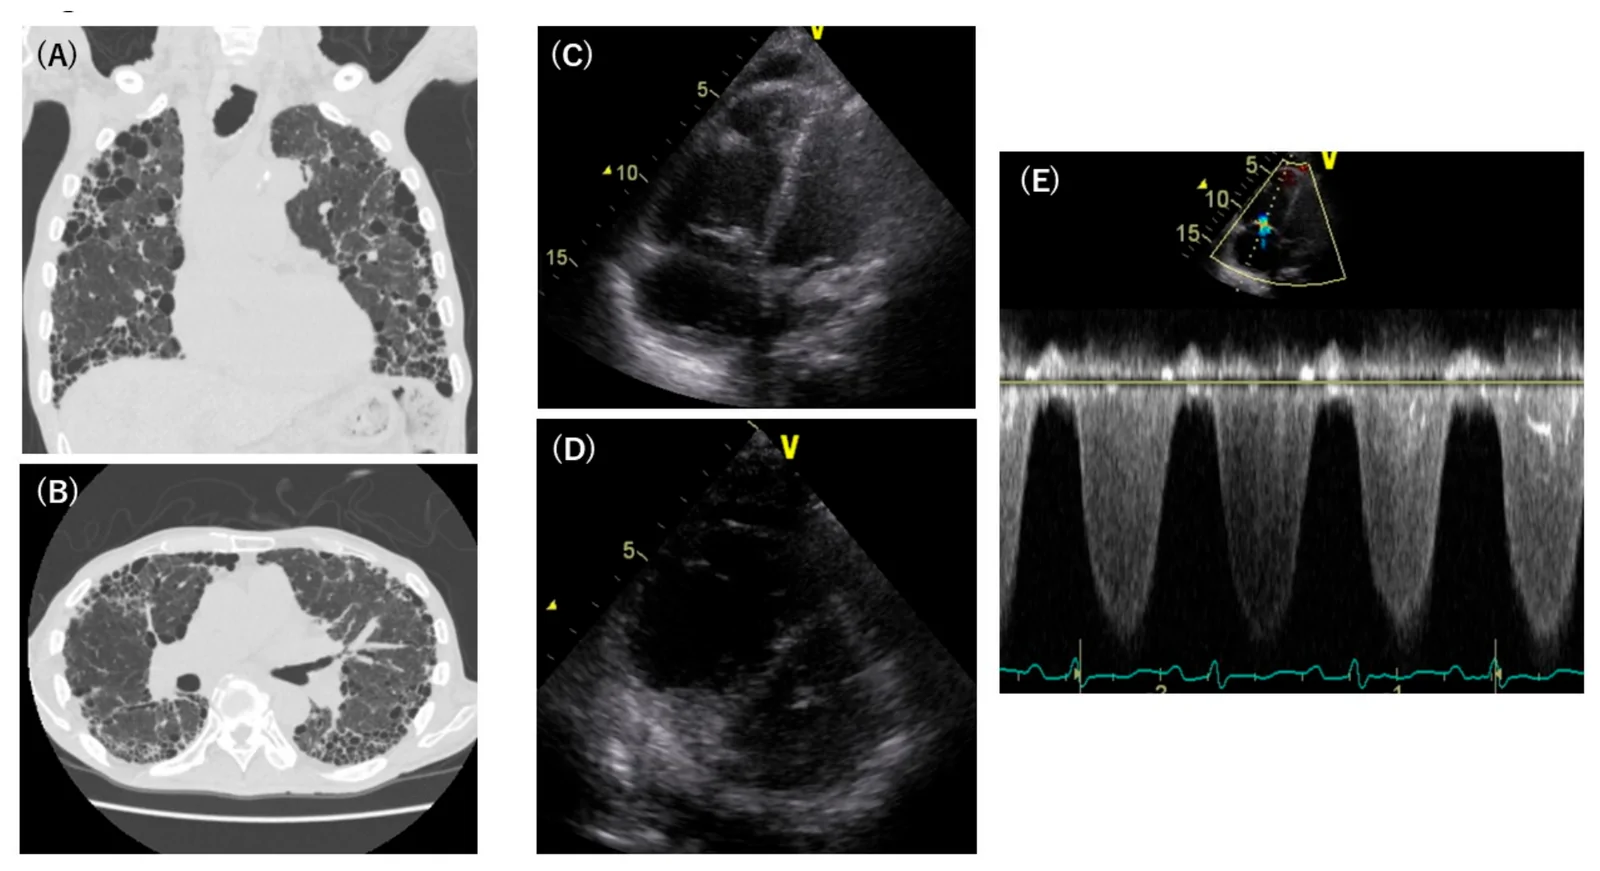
Representative chest HRCT and echocardiographic images of a patient with severe interstitial lung disease and pulmonary hypertension (ILD-PH). (A) Coronal and (B) axial views of chest HRCT demonstrating extensive fibrotic changes and honeycombing predominantly in the lower lobes. (C) Apical four-chamber view on transthoracic echocardiography showing severe right ventricular dilation and a leftward shift in the interventricular septum, indicating right heart volume and pressure overload. (D) Parasternal short-axis view at the papillary muscle level revealing a D-shaped left ventricle, consistent with severe right ventricular pressure overload (ventricular interdependence). (E) Continuous-wave Doppler of the tricuspid regurgitation jet showing a high-velocity signal (TR Vmax = 3.6 m/s, yielding a pressure gradient of 52 mmHg), used to estimate the markedly elevated right ventricular systolic pressure (RVSP).
